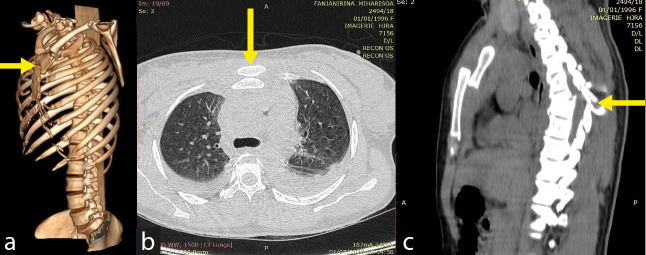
(a) Left sagittal view of 3D CT scan showing manubriosternal joint dislocation type
